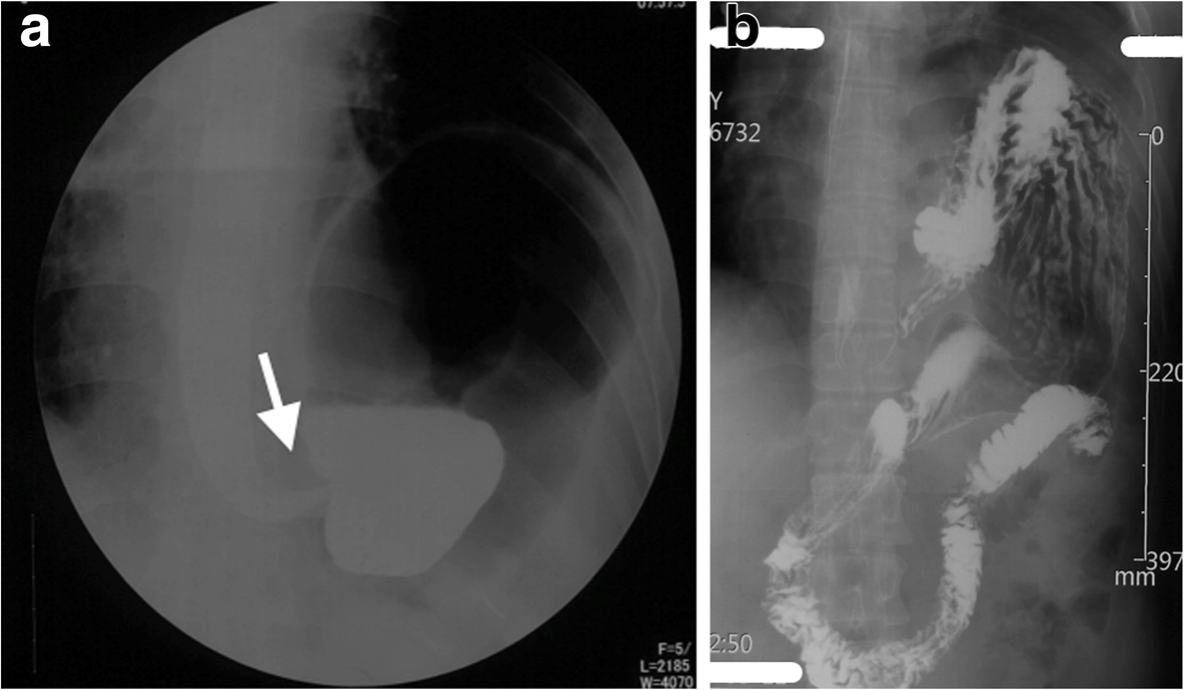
Upper digestive tract radiograph. a showed gastric volvulus with vertical gastroesophageal angle (the arrow). b showed the full view of the gastric volvulus
